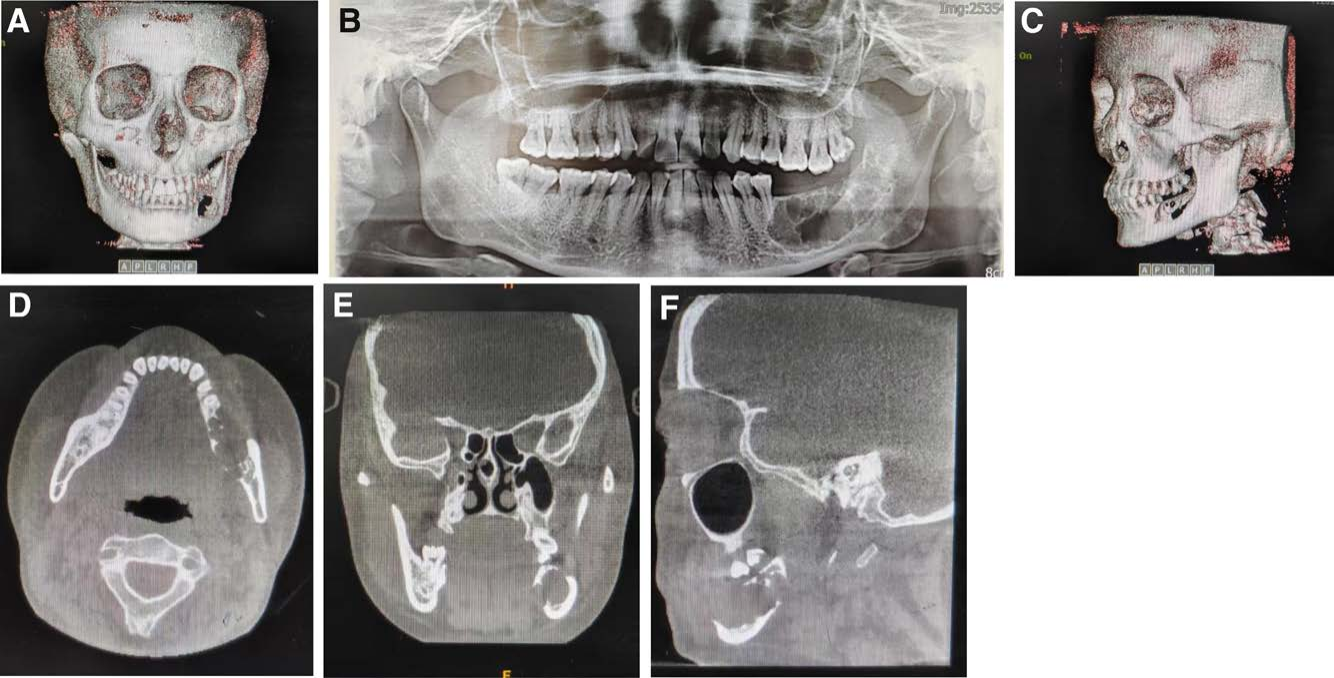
The surface tomography and CT image manifestations of patient. (A) Preoperative frontal image of the jawbone. (B) The preoperative surface tomographic images of patient. (C) Preoperative lateral image of the jawbone. (D) Horizontal scan manifestation. (E) Coronal scan manifestation. (F) Sagittal scan manifestation.
